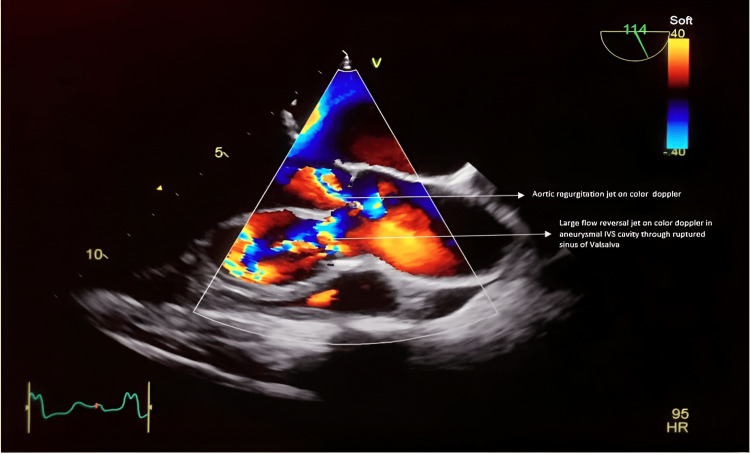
Two-dimensional transesophageal echocardiography imaging on color Doppler highlights regurgitation jets observed in the left ventricular outflow tract and within the aneurysmal cavity in the interventricular septum.
